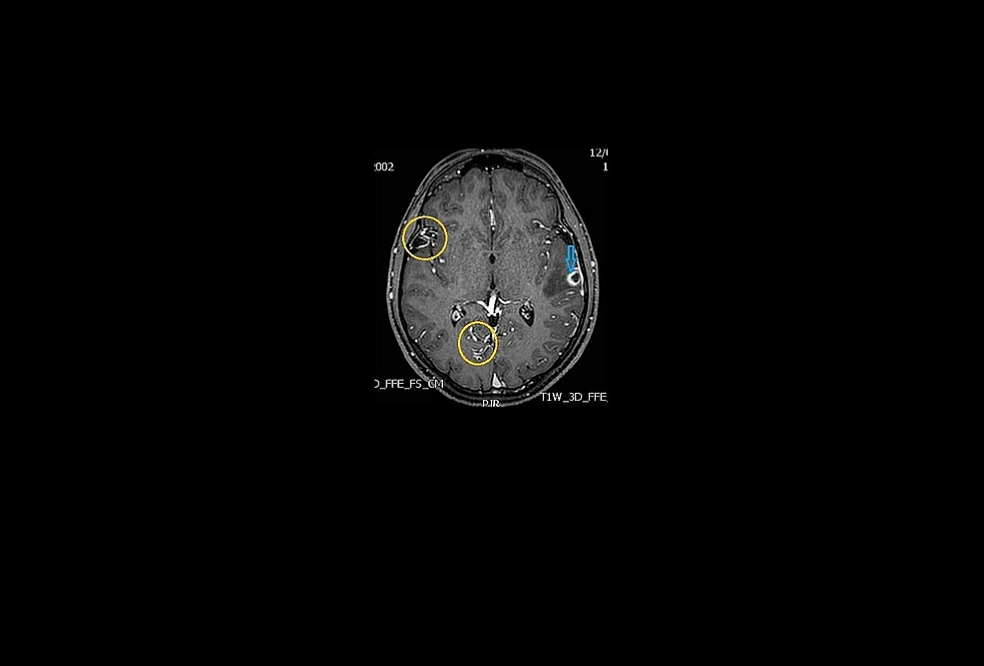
Axial images of T1 weighted MRI of brain arterionenous malformations (yellow circles). The blue arrow shows the brain abscess’s area.
